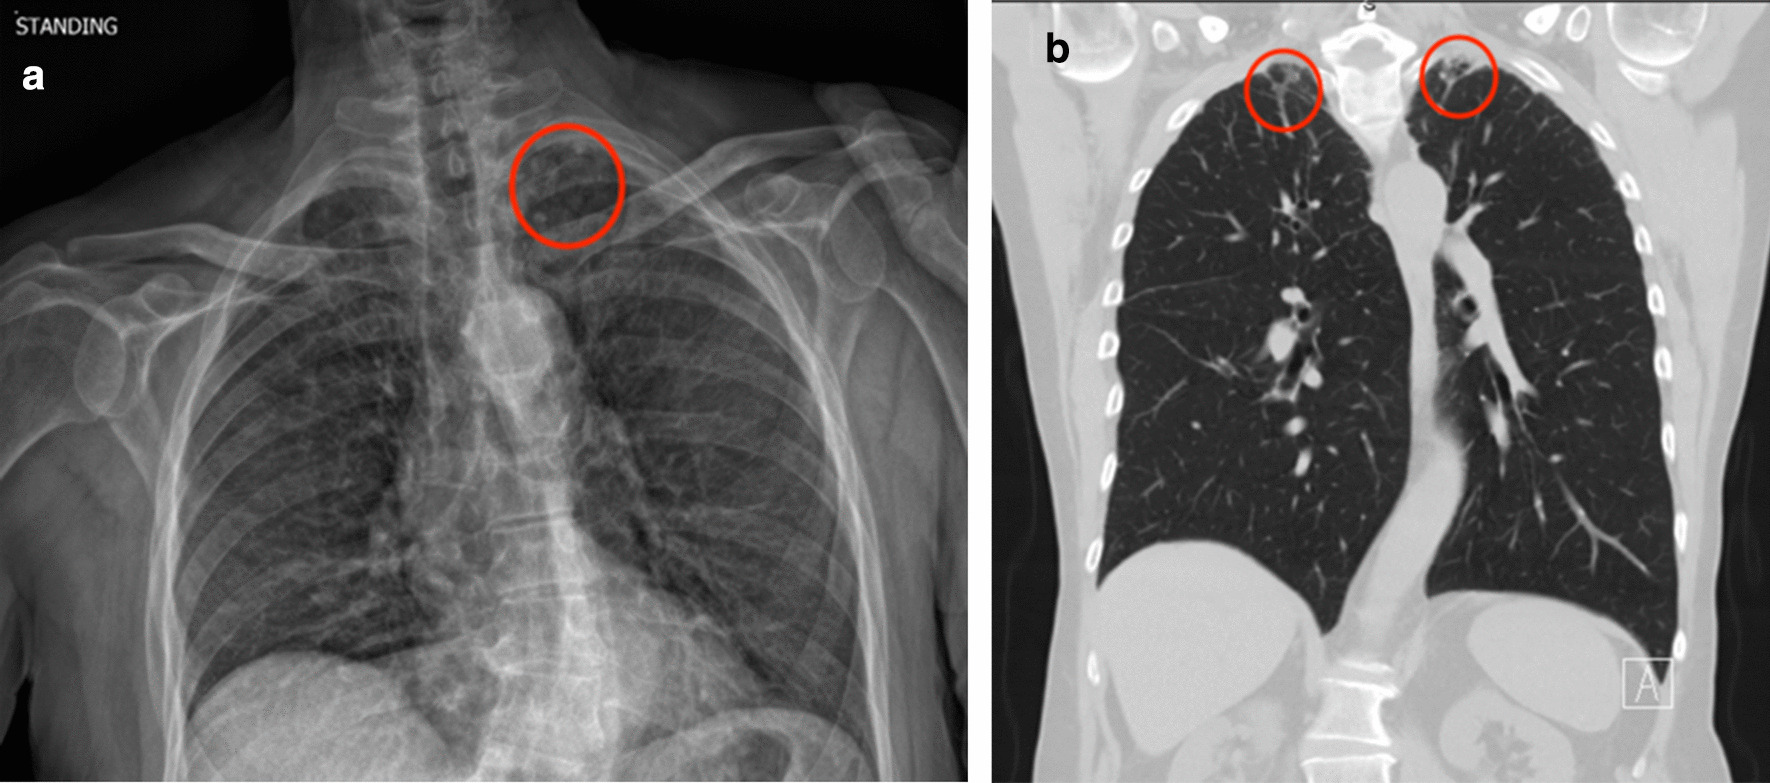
a A 70-year-old man with 4–5 mm left apical pulmonary nodules (circle) (a *Moderate* finding) on EOS imaging. b The same patient with subsequent coronal reconstruction of CT chest using a lung window showing bi-apical subpleural reticulonodular opacities (circles), raising concern for possible interstitial lung disease (ILD)
